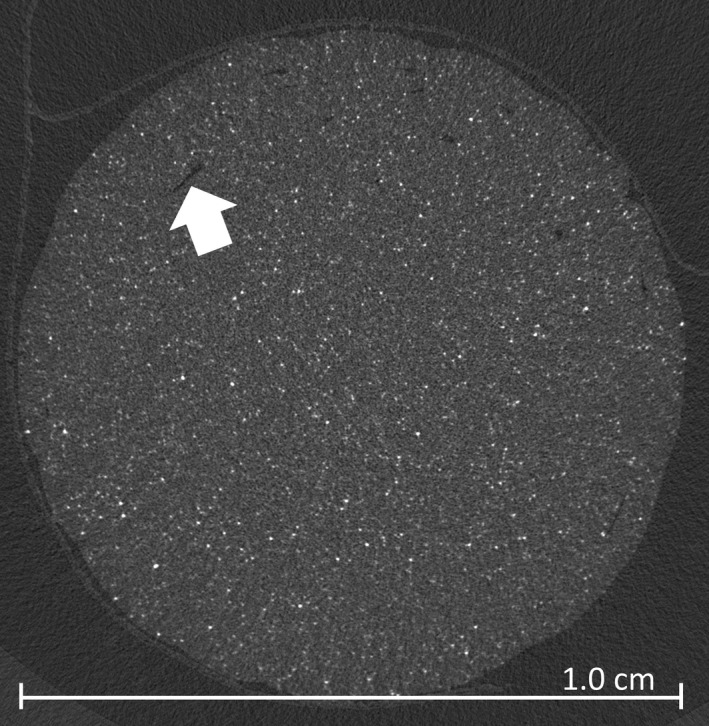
Micro‐CT image of a 5% doped, 1.0 cm cylinder model. Small air pockets are evident (arrow) but infrequent. HU value measurement of ten sampled regions (2.5 mm diameter) yielded a standard deviation that was 5.7% of the average mean.
